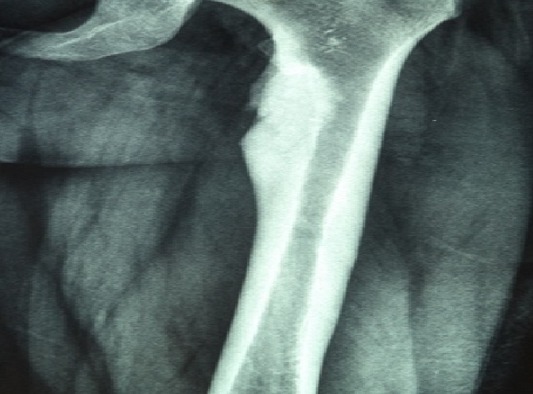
Flowing candle wax condensation in the proximal femur on a plane radiography, specific of the Leri's disease
